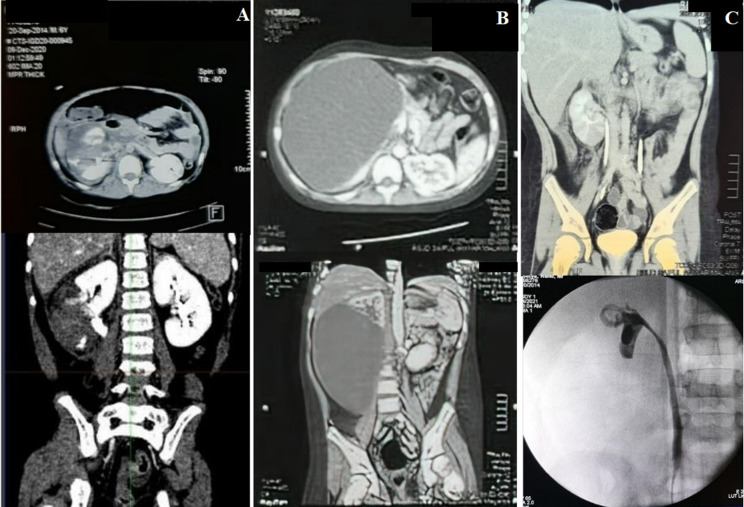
evaluation and clinical findings in the second patient A) initial CT scan showed right kidney laceration involving the pelvicalyceal system, segmental artery injury with leakage of urine in perirenal: coronal view (upper), axial view (lower); B) 1-week urgent CT scan evaluation, large urinoma in right kidney: coronal view (upper), axial view (lower); C) abdomen CT scan evaluation 1 month after to urinoma drainage (upper), RPG evaluation during DJ Stent removal (lower)
